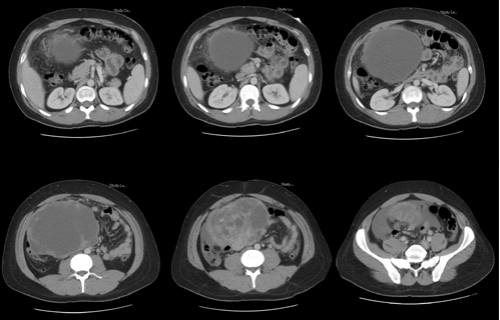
CT images at six different levels demonstrates a large 22 cm intraabdominal mass displacing the small bowel to the left.
